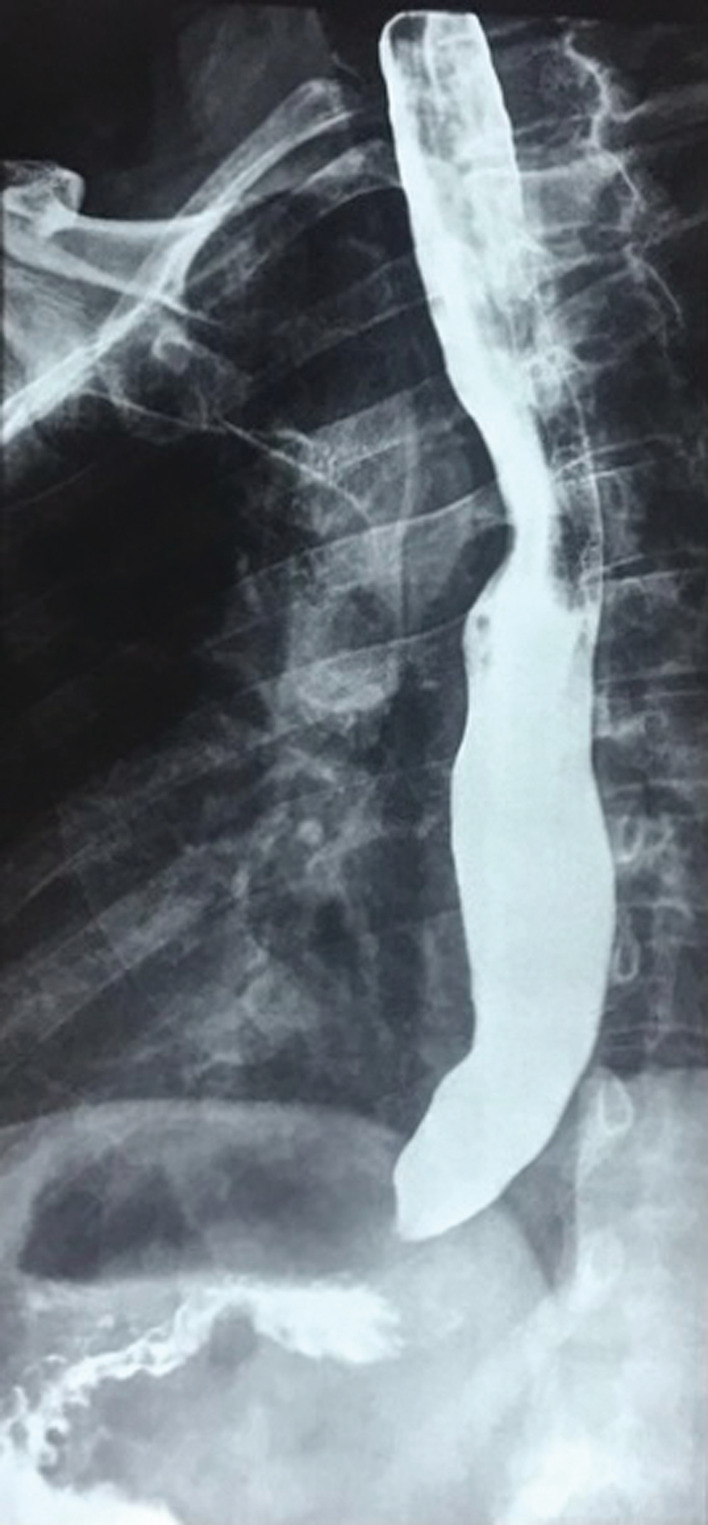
Upper gastrointestinal radiograph after barium swallow showing a typical “bird‐beak” view indicative of achalasia
